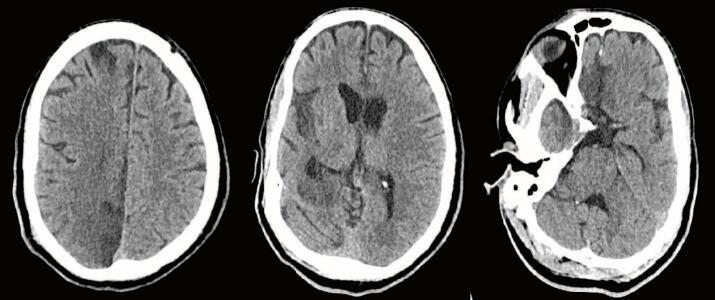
CT Head two months after discharge CT Head two months after injury showing resolution of the SDH with evolving encephalomalacia of right frontal and occipital lobes.
